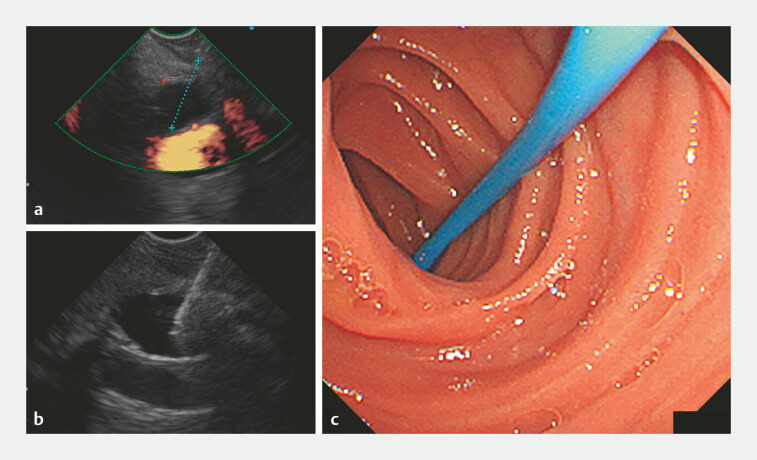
Endoscopic ultrasound-guided hepaticojejunostomy (EUS-HJS) using a direct-view convex EUS.
